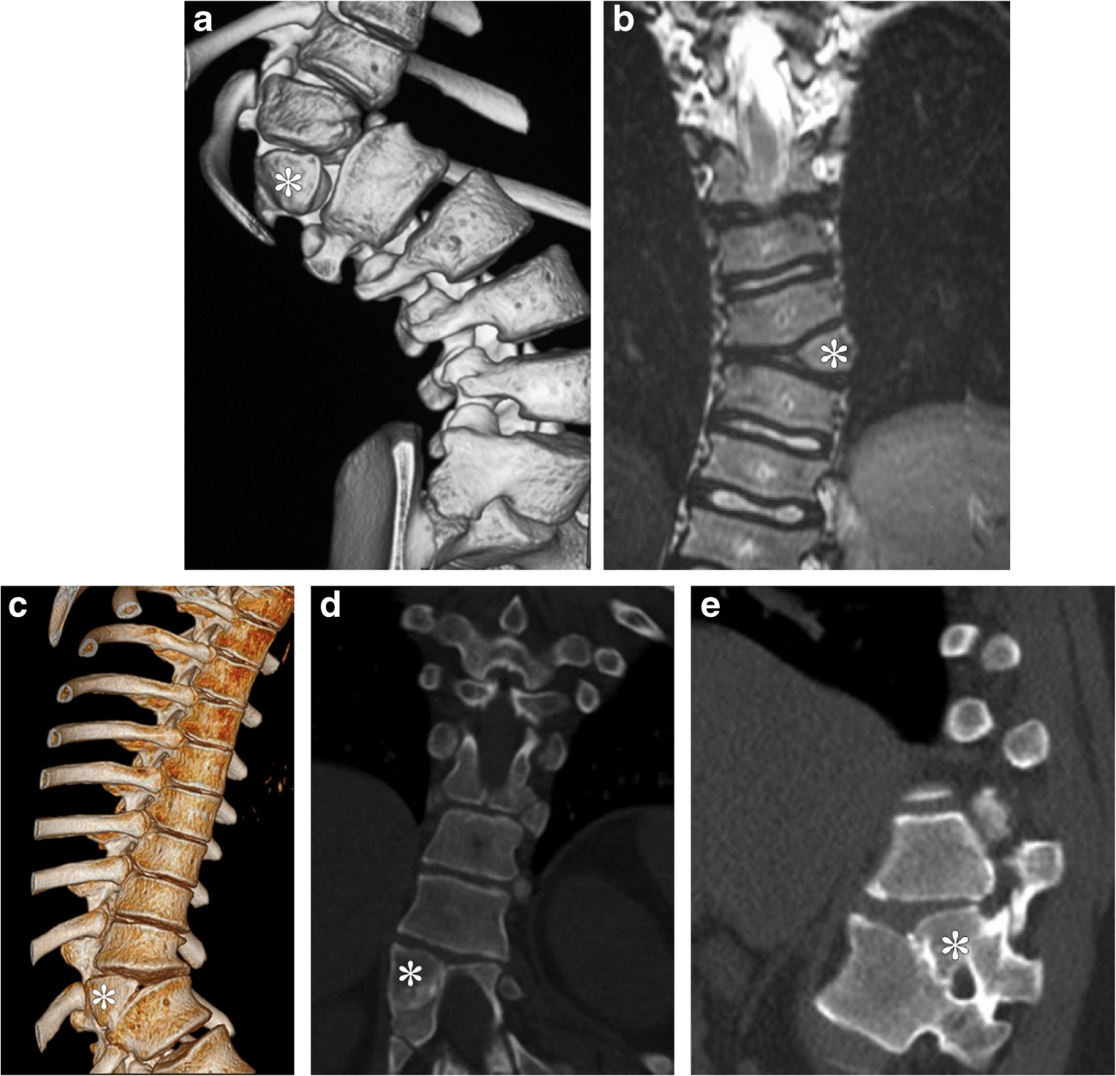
a-b: Segmented hemivertebra in two patients: The 3D reconstruction from a non-contrast CT of the spine (*a*) and coronal T1-weighted MR image of different levels of the thoracic spine (*b*) demonstrate a laterally located wedge of bone composed of half a vertebral body (marked by *asterisks*). Note that there is associated scoliotic deformity, more pronounced on *a*. This variety of hemivertebra, being clearly separate from its neighbour above and below, has the most potential for impacting spinal curvature. c-e: Semisegmented hemivertebra in 16 year old: 3D reconstruction (*c*) and coronal and oblique sagittal reconstructions from a non-contrast CT of the spine (*d* and *e*) demonstrate a laterally located wedge of bone composed of half a vertebral body (marked by asterisks). Note that this variant is attached to its neighbouring inferior vertebra and has only the superior disc space adjacent to it
